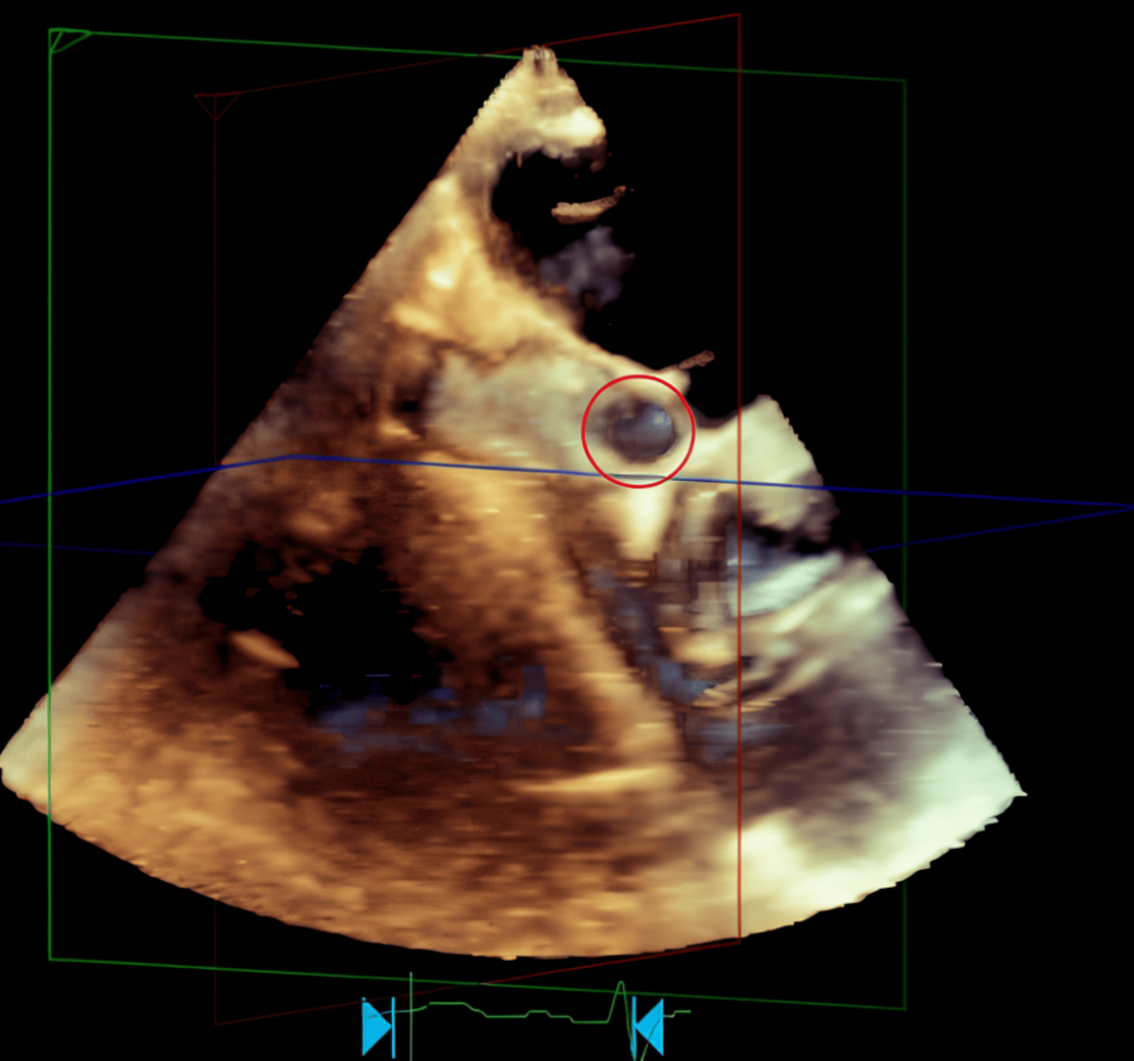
Secundum ASD-TEE 3D view Right atrial en face view showing secundum ASD (red circle) ASD: atrial septal defect; TEE: transesophageal echocardiography
